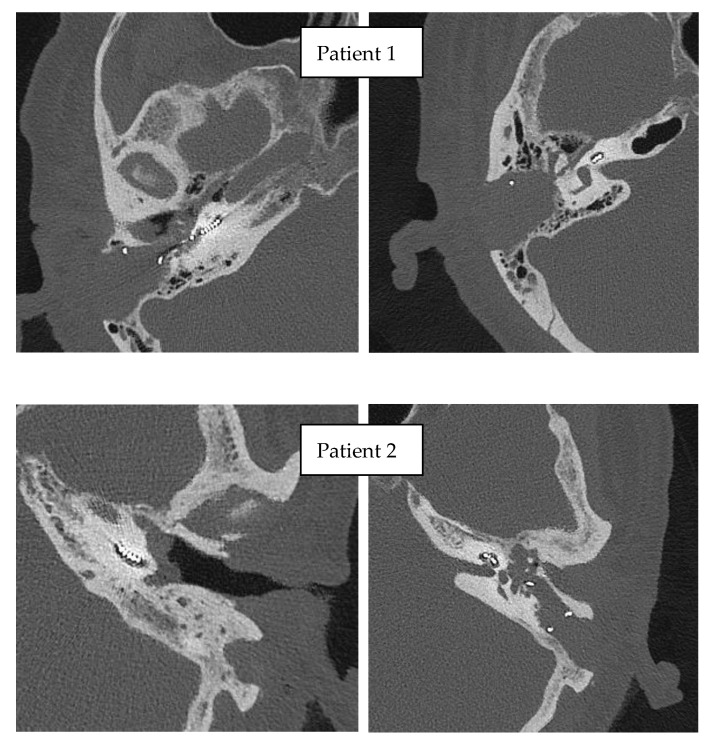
Postoperative CT scans of operated patients.
